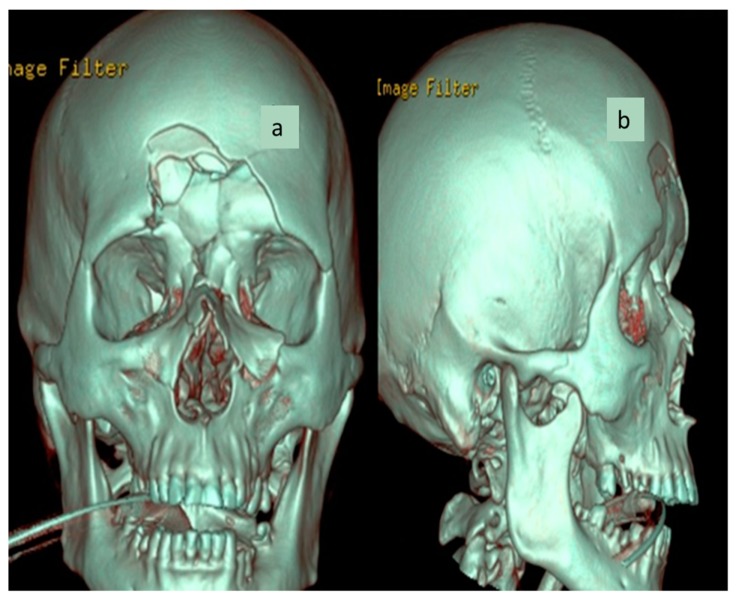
(a) Three-dimensional CT reconstruction—coronal. (b) Three-dimensional CT reconstruction—sagittal.
